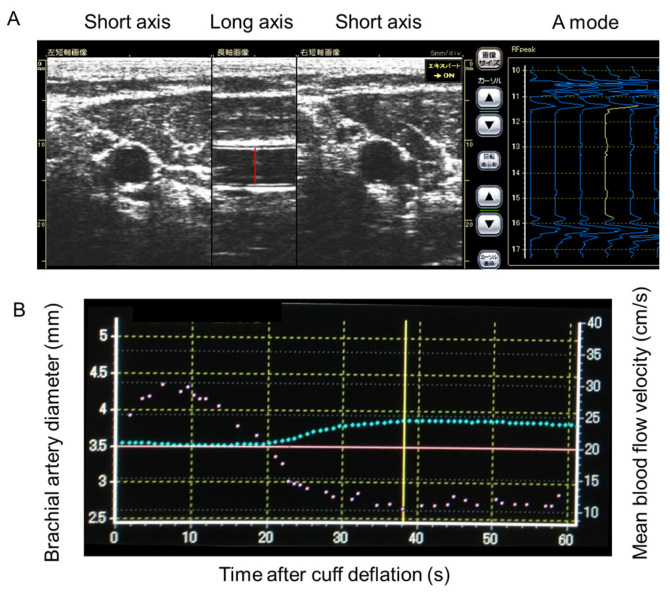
Assessment of flow mediated dilation (FMD) with UNEXEF18G. (A) The H-shaped probe of the UNEXEF18G shows 2 short-axis and 1 long-axis images of the brachial artery (left) and both the near and far walls of the brachial artery as A-mode ultrasound signals (rt). The red line indicates diameter of the brachial artery. (B) Representative images of the change in brachial artery diameter after cuff deflation to measure FMD. The green dot line and red dot line indicate the changes in brachial artery diameter and blood flow velocity, respectively. FMD is measured at the point indicated by the yellow line.
